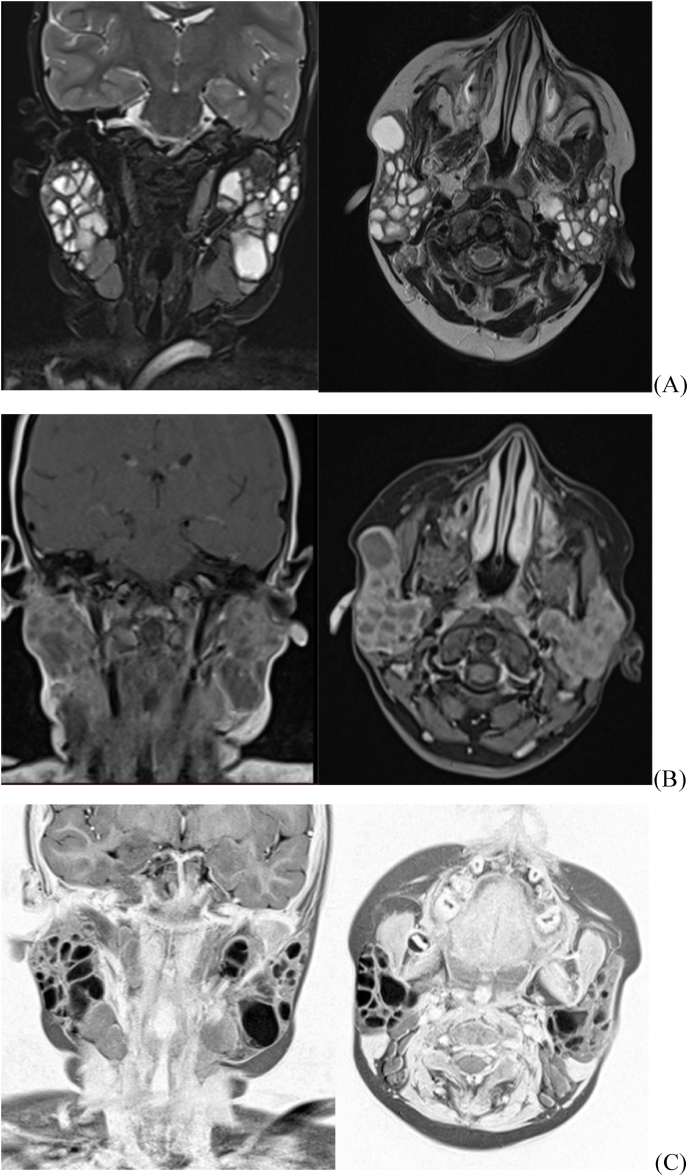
MRI pictures axial and coronal T2-weighted (A) T1-weighted (B) negative T2-weighted (C) showing enlarged parotid glands with multi-loculated cystic formations, the septa are of intermediate signal enhancing after gadulinium injection.
